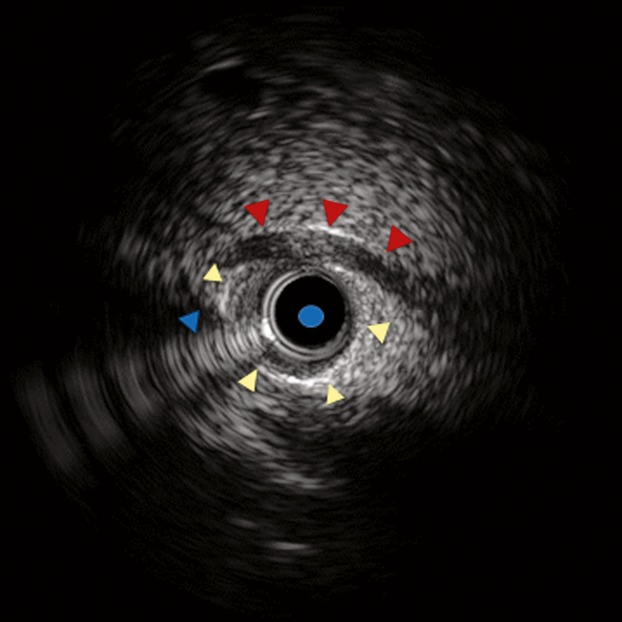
Intravascular ultrasound (IVUS) picture of an echo‐lucent half‐moon sign. The red arrows outline the echo‐lucent half‐moon sign. The yellow arrows outline the vessel wall. The blue round circle is positioned in the center of the IVUS catheter and the blue arrow points to an artifact from the catheter.
